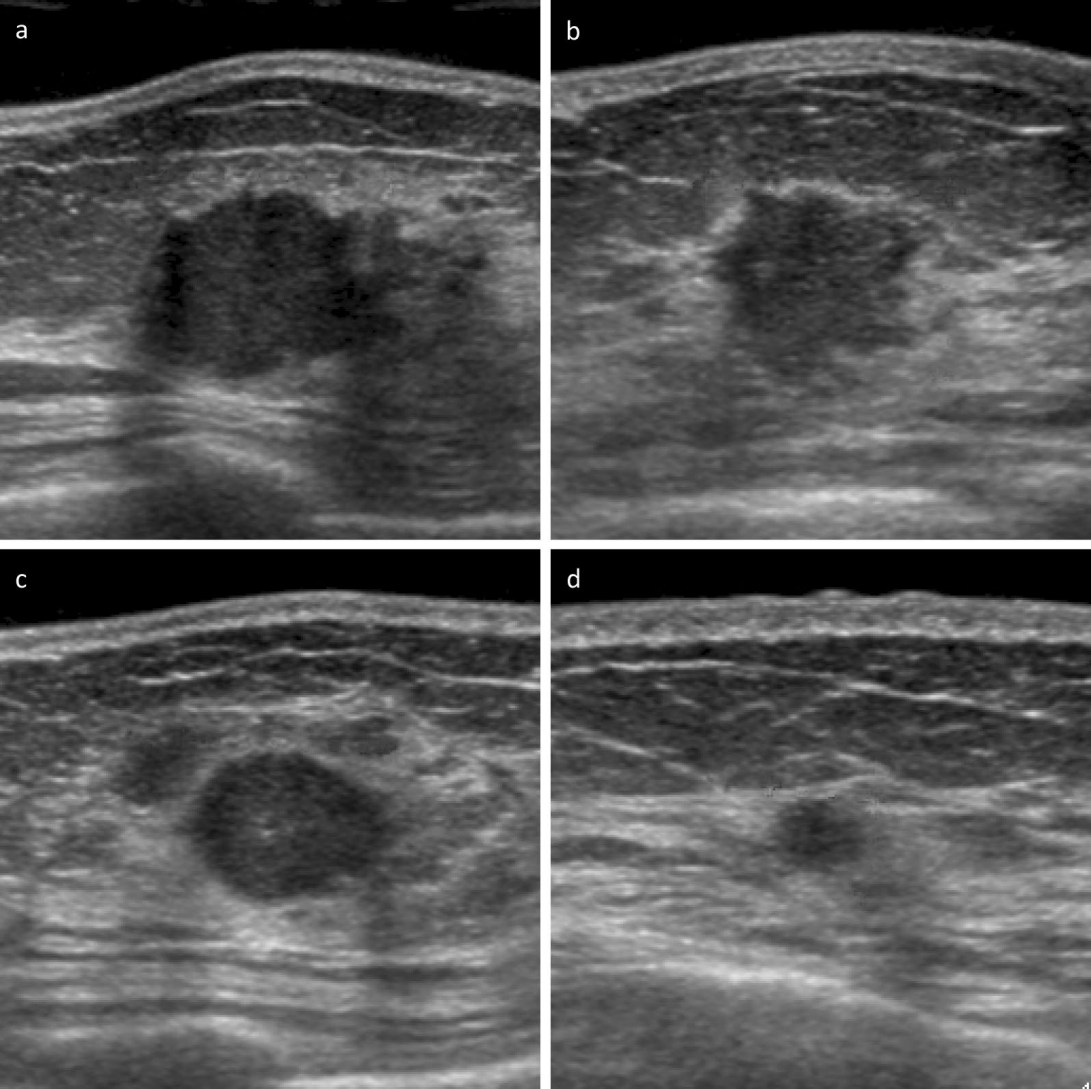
Ultrasonographical findings of the breast tumors. Multiple low echoic tumors with irregular margins in both breasts were observed. a The breast tumor in the right upper-outer area (1). b The breast tumor in the left lower-inner area (2). c The breast tumor in the left upper-outer area (3). d The breast tumor in the left upper-inner area
